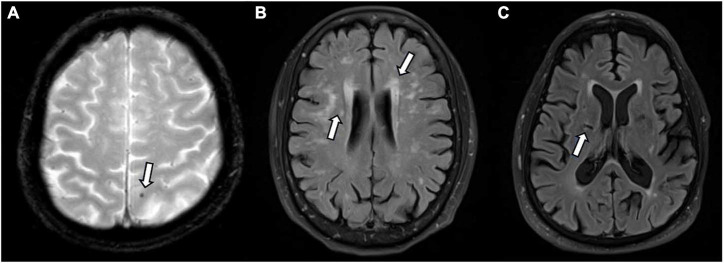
Small vessel disease as identified on magnetic resonance imaging (MRI) by white arrows. (A) Microbleed identified on T2*; (B) white matter hyperintensities identified on FLAIR; (C) lacune on FLAIR.
